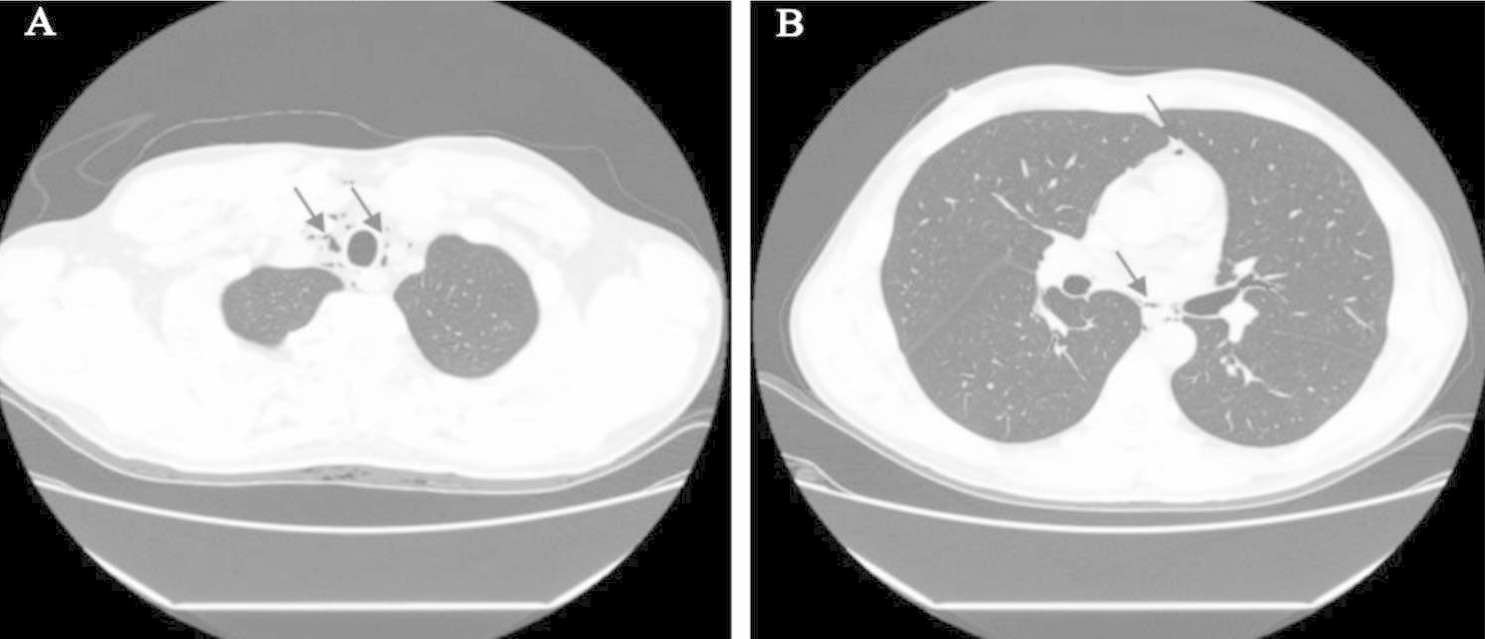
Chest Computed tomography-scan showed air streaks distributed from the paratracheal parenchyma to the pericardium (black arrow)
